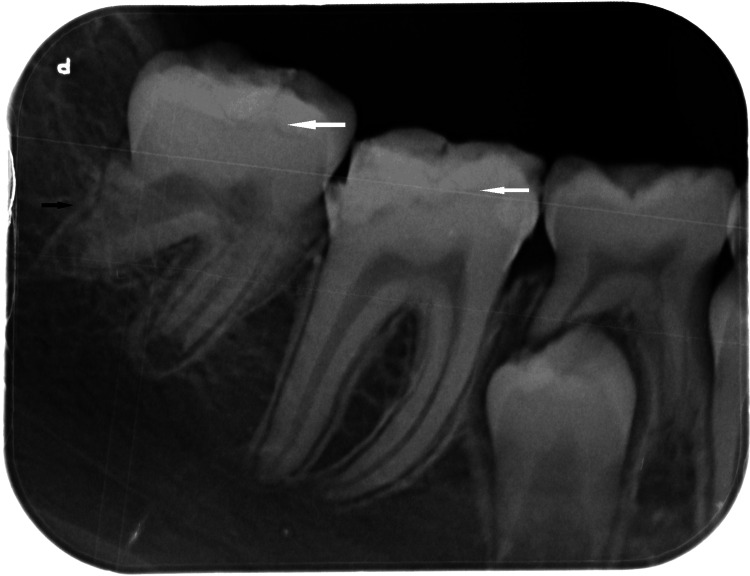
Long-term radiographic outcome showing eruption of the mandibular right second molar despite altered root development Periapical radiograph showing hypomineralized enamel affecting teeth 46 and 47 and an abnormal distal root morphology of tooth 47 following conservative management. White arrows indicate the enamel defects, while the black arrow highlights the divergent distal root of tooth 47.
